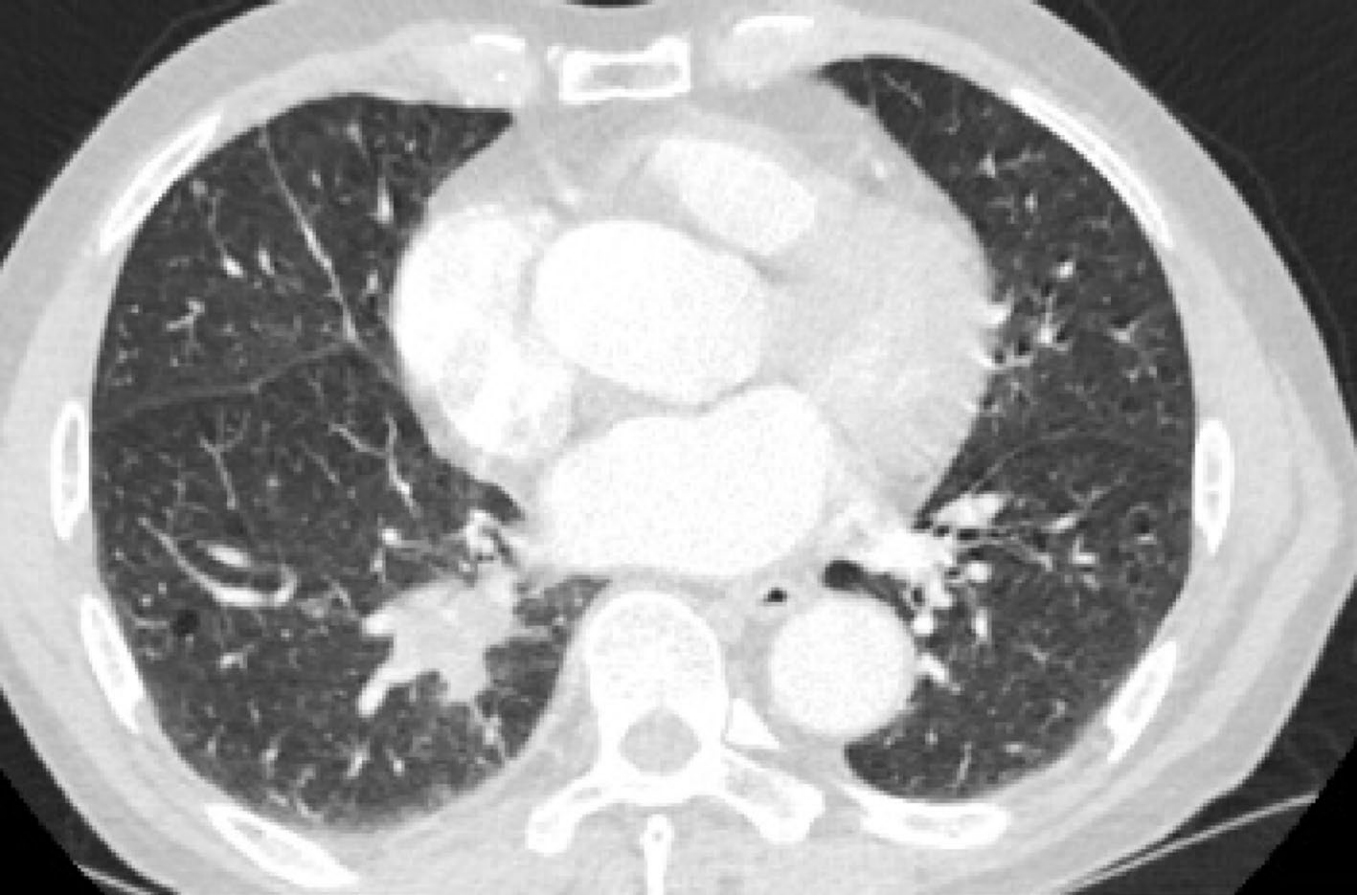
Case2 23 September 2024 CT
